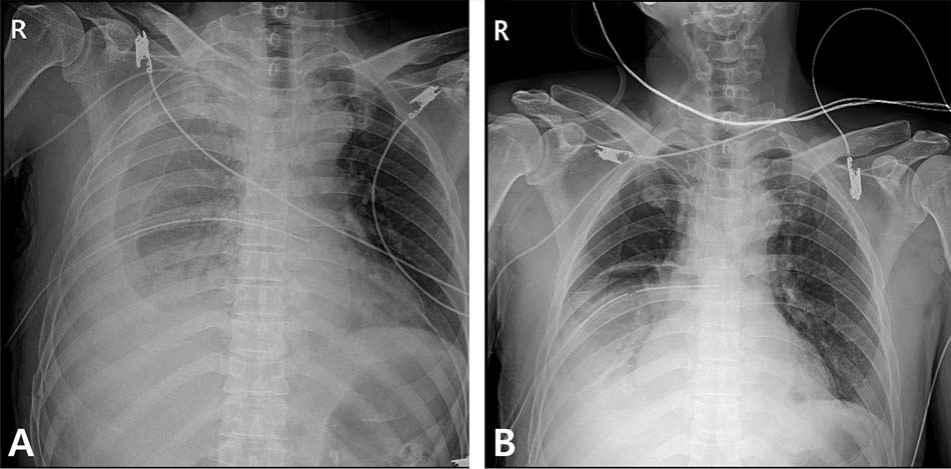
Radiographic findings. (A) After chest tube insertion. (B) 12 h after chest tube insertion.
